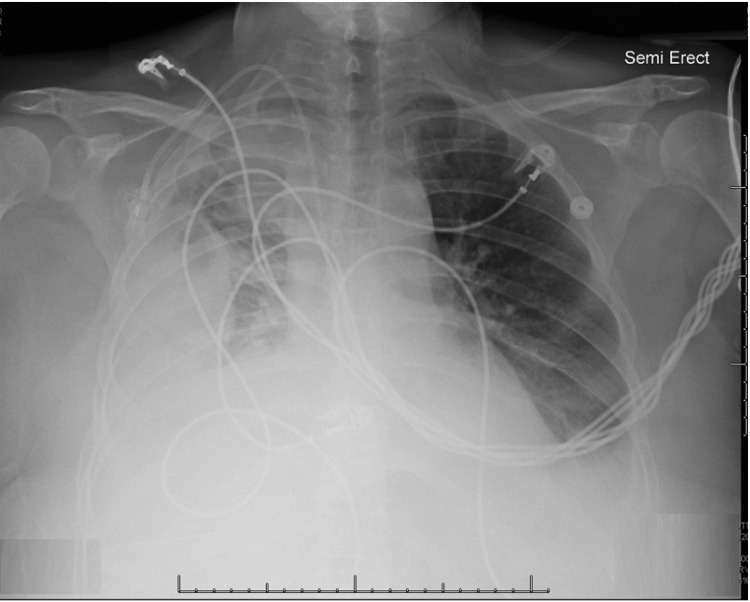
A chest X-ray showing a right lobe pleural effusion with near complete right-sided opacification, without a definitive pneumothorax. A much smaller pleural effusion is also seen on the left lung. Both lungs showed stable lucencies consistent with known pneumatoceles.
